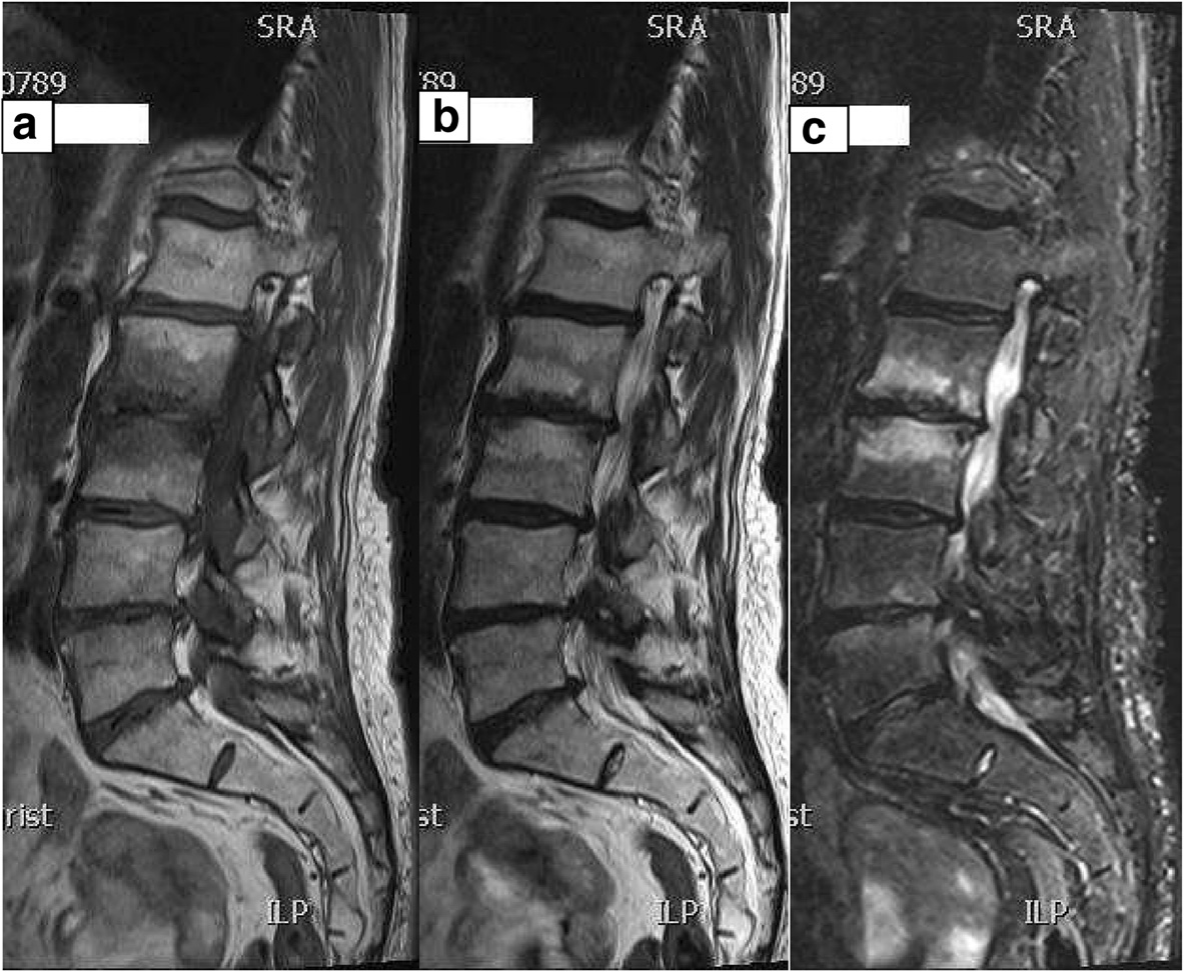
a, b and c Modic type I changes. T1, T2 weighted and fat suppressed sagittal MR images of a patient demonstrating low signal intensity adjacent to L2-3 disc on T1 weighted images (2a) and high signal intensity on both T2 weighted image (2b) and fat suppressed image (2c), consistent with MC type I (marrow edema) at L2 through 3
